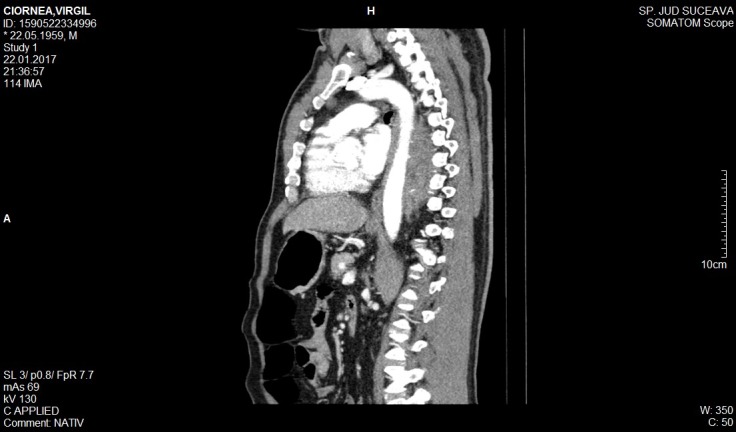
Thoracoabdominal CT - “coated aorta”
